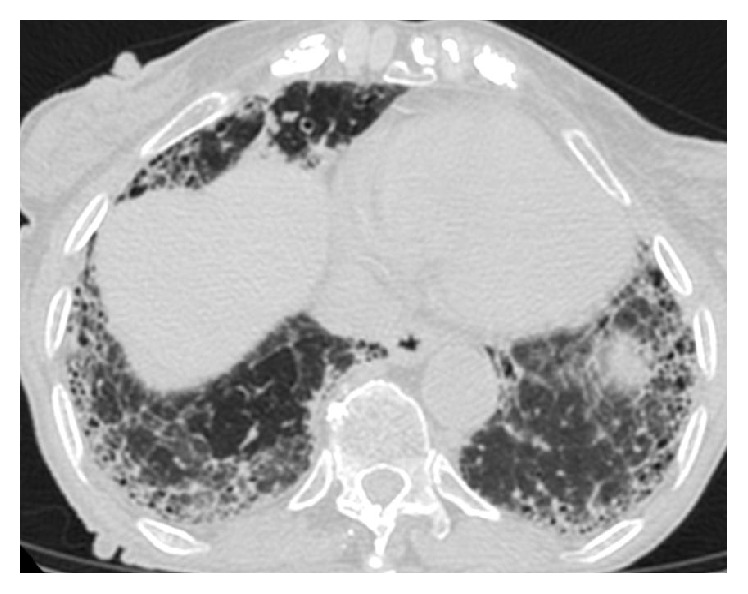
HRCT of the chest at the level of the lower lobes. An extended and coarse subpleural reticular pattern is noted associated with traction bronchiectasis and bronchiolectasis as well as with honeycombing.
